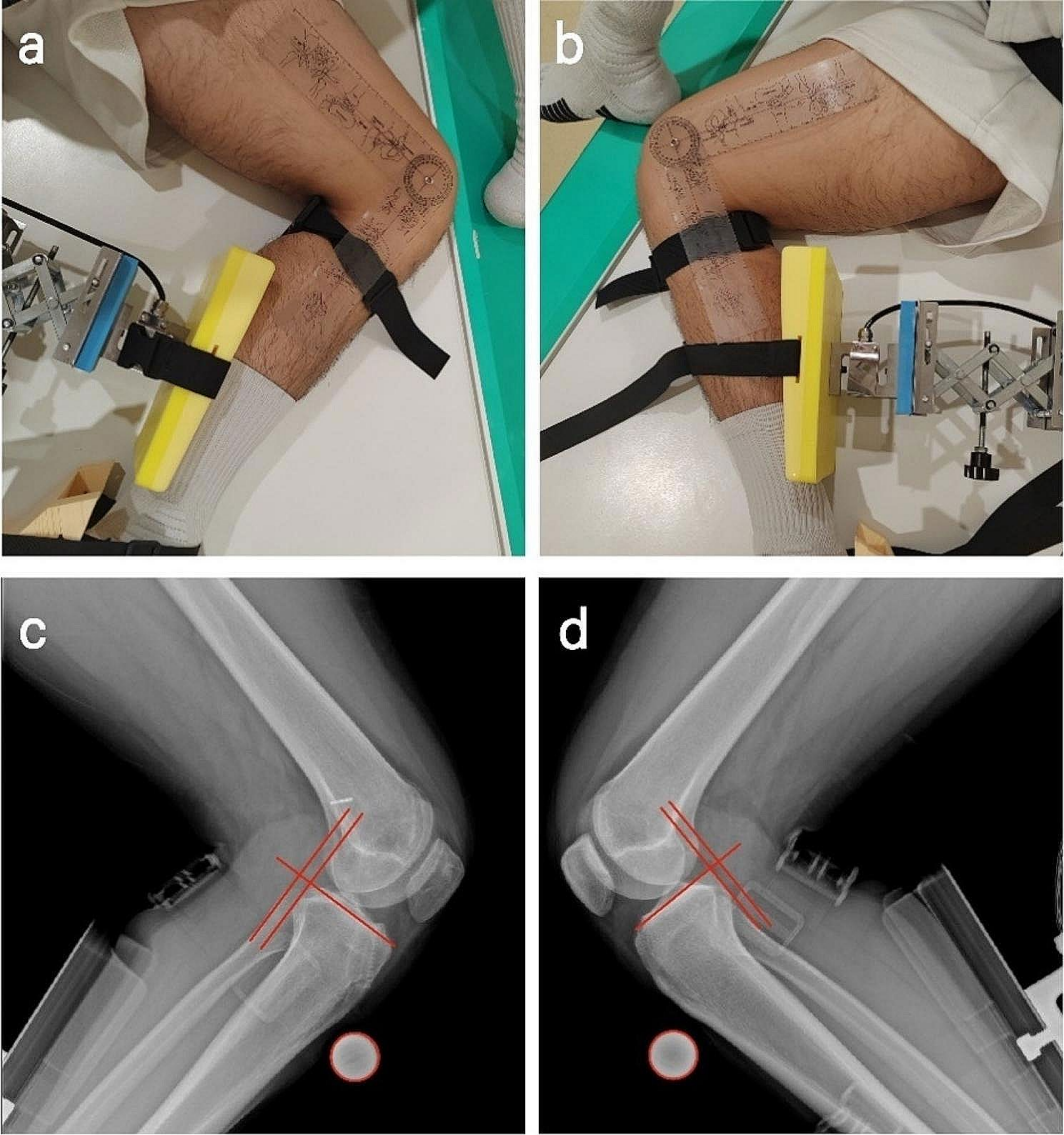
Stress radiographs were taken at 90° flexion: (a)left and (b)right knee. Perpendicular lines from the reference line (parallel to the medial tibial plateau joint line) were drawn tangentially to the posterior contour of the medial femoral condyle and the posterior contour of the medial tibial plateau. The distance between the medial femoral condyle and the medial tibial plateau was measured to calculate the side-to-side differences: (c) and (d)
